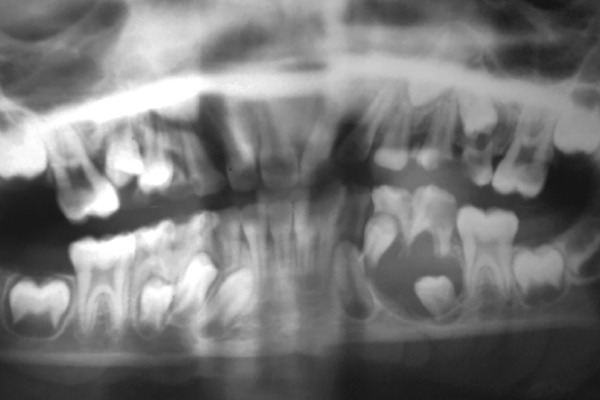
Panoramic radiograph showing a unilocular radiolucency enveloping the unerupted left mandibular second premolar
